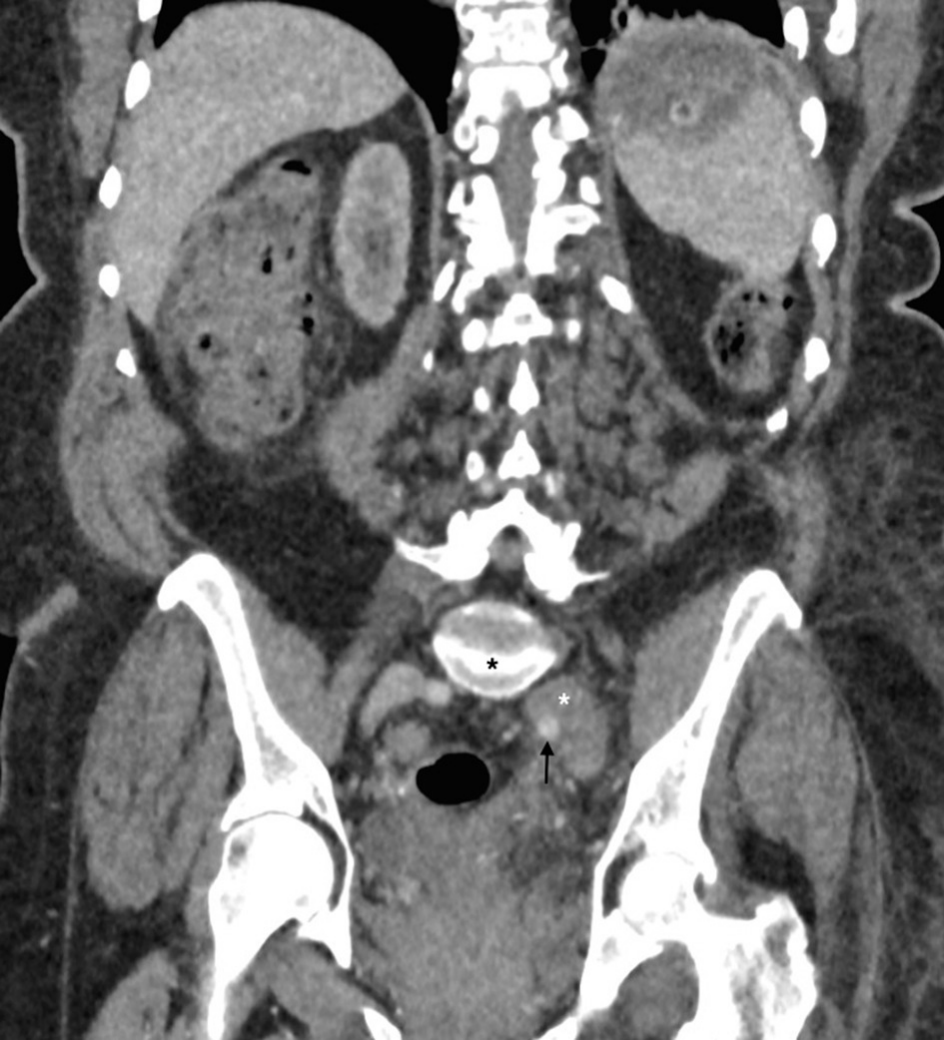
Coronal reconstruction CT demonstrating compression of the left common iliac vein between the right common iliac artery (black arrow) and anteriorly translated lumbar spine (black asterisk). Low attenuation thrombus is seen within the expended left external iliac vein (white asterisk). Note left-sided soft tissue oedema secondary to deep venous thrombosis.
